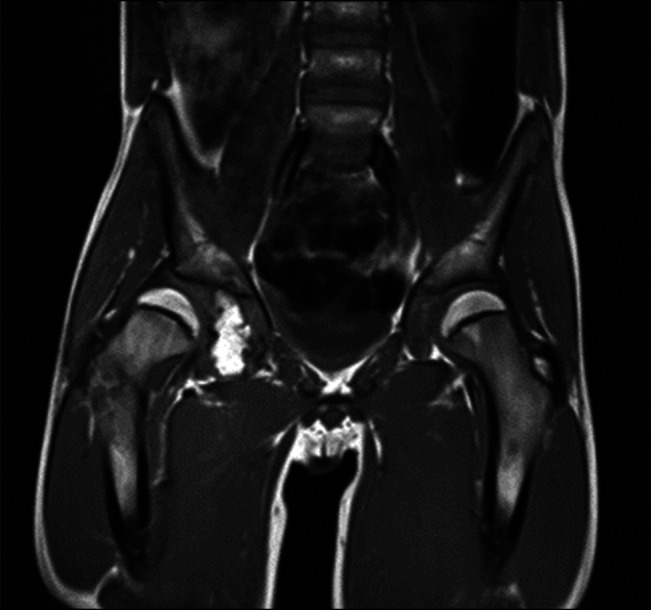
MRI of the pelvis showing osseus abnormality involving the base of the right acetabulum and extending to the triradiate approximately 5.5 × 2.5 cm confluent with base of acetabulum. Minimal cartilage cap.
